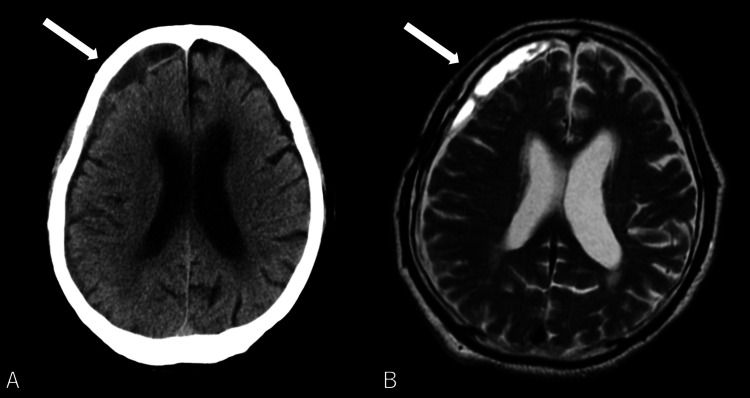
Brain computed tomography scan (A) and magnetic resonance imaging (B) showing a chronic subdural hematoma in the right frontal region (arrows).
